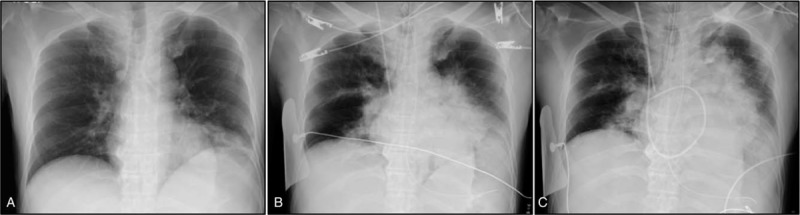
An initial chest X-ray revealed infiltration in the left lower lobe of the lung (A). Follow-up chest X-rays revealed a rapid progression to voluminous hyperdense lung infiltration with bulging interlobar fissure in the left lung and developing multiple patchy infiltrations in the right lung (B, 9 hours after admission and C, 11 hours after admission).
